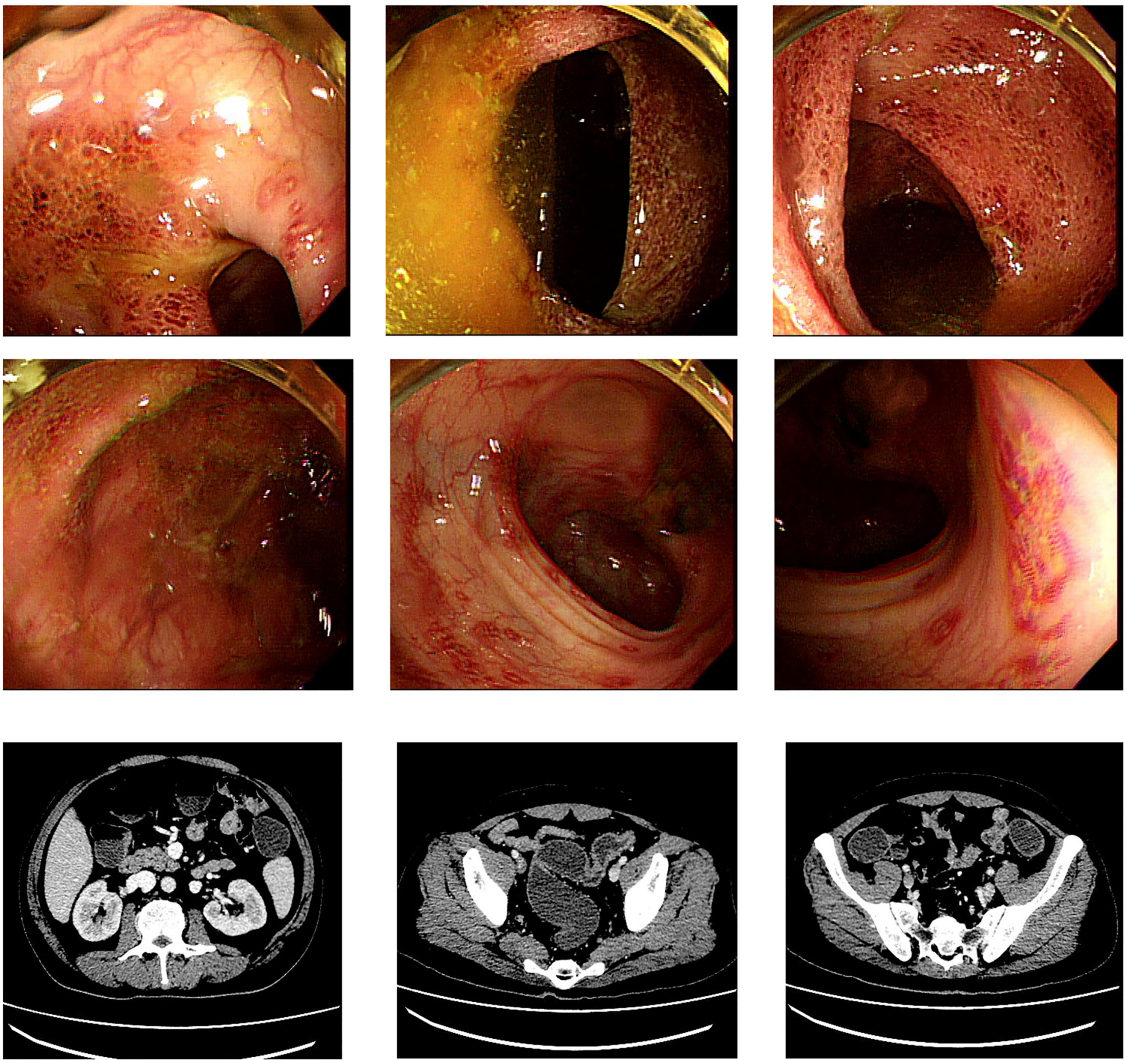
Colonoscopy and CT manifestations at diagnosis: Colonoscopy: extensive erythema, edema, fragility, loss of blood vessel texture, erosion and ulcers in the colorectal; CT: colorectal dilatation, effusion, visible air-fluid level.
